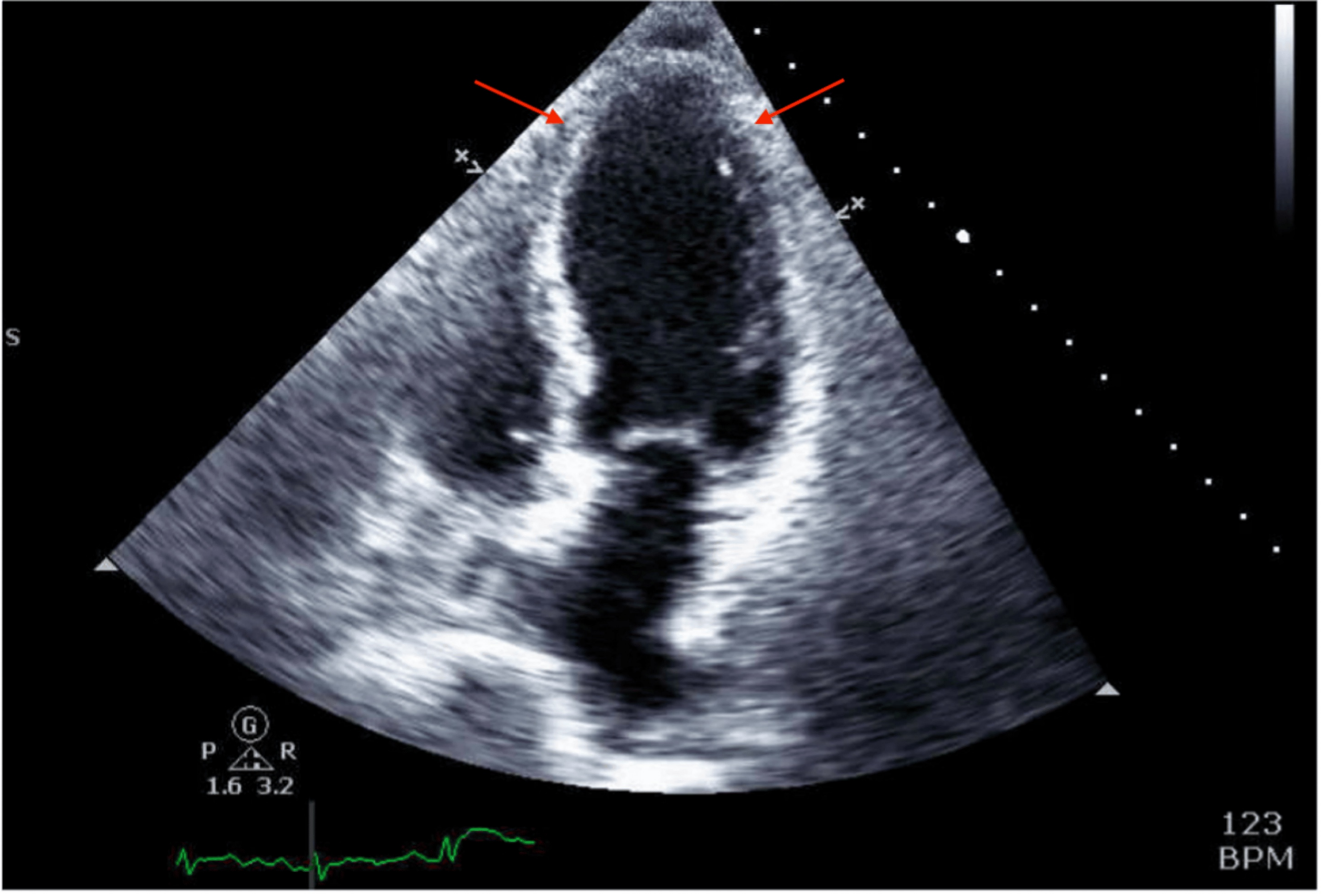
TTE in apical four-chamber view demonstrating LV apical hypokinesis Red arrows indicate the apical region of the left ventricle, which exhibits reduced wall motion consistent with apical hypokinesis. TTE: transthoracic echocardiogram, LV: left ventricular, BPM: beats per minute
